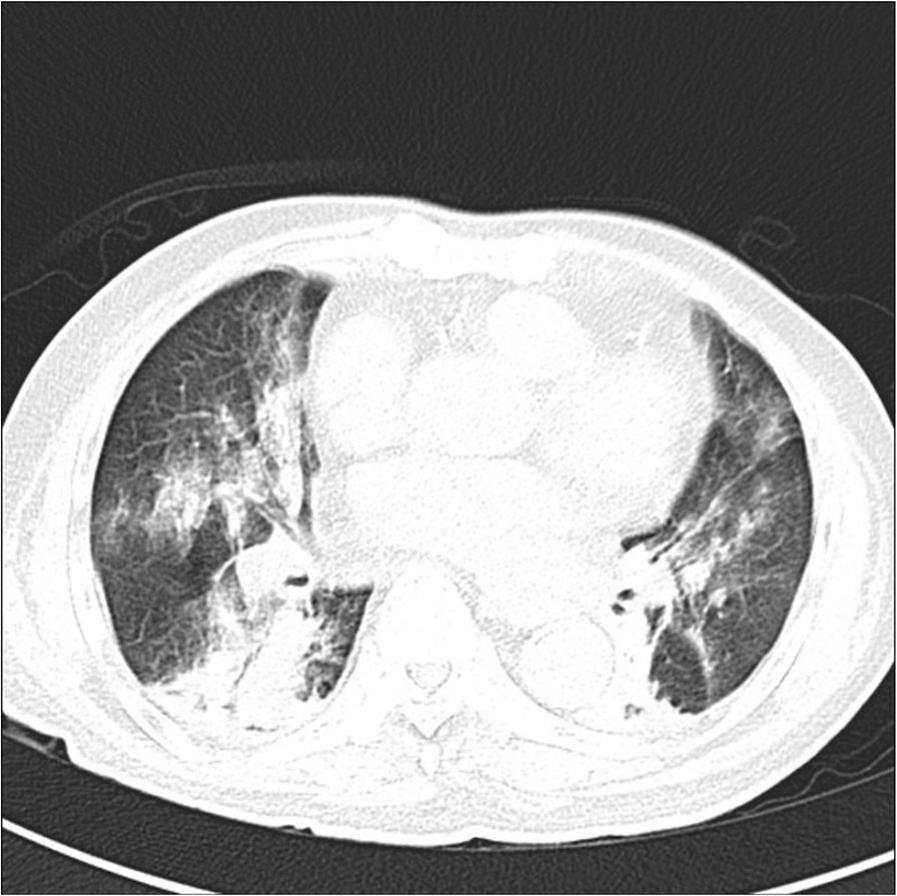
Chest computed tomography scan showed diffuse pneumonia in both lungs with multifocal ground-glass opacities and crazy paving patterns
